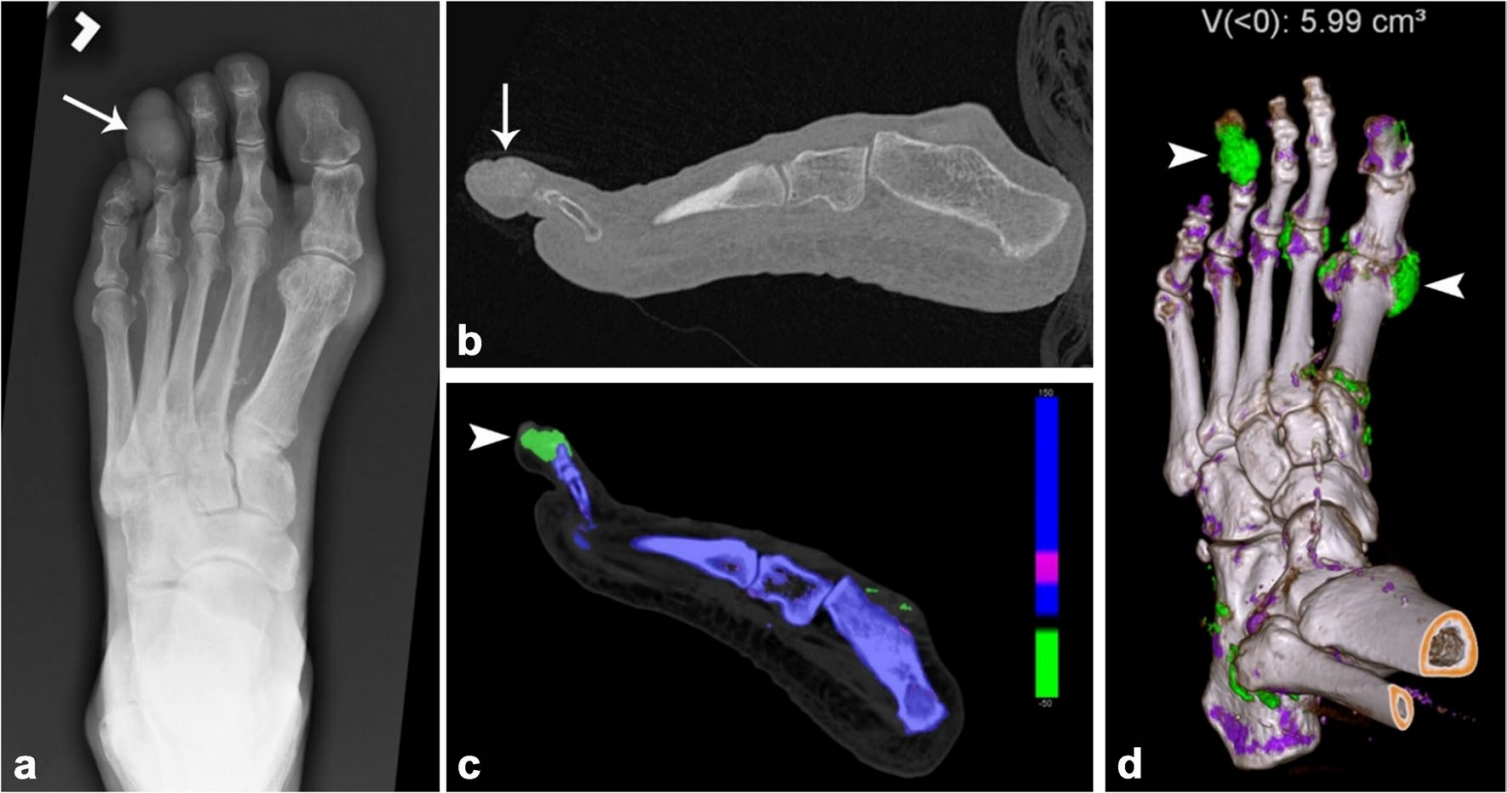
Eighty-five-year-old man with left fourth toe pain and swelling. AP radiograph (a) of the left foot demonstrates osseous destructive change of the fourth middle and distal phalanges (*arrow*), which could be mistaken for osteomyelitis. Dual-energy CT sagittal reformation (b), color map (c), and color-coded 3D reconstruction (d) demonstrate the gout (green, *arrowheads*) deposition, including intraosseous gout corresponding to the lytic areas (*arrow*). The calculated volume of monosodium urate deposition of the left foot was 5.99 cm.^3^
